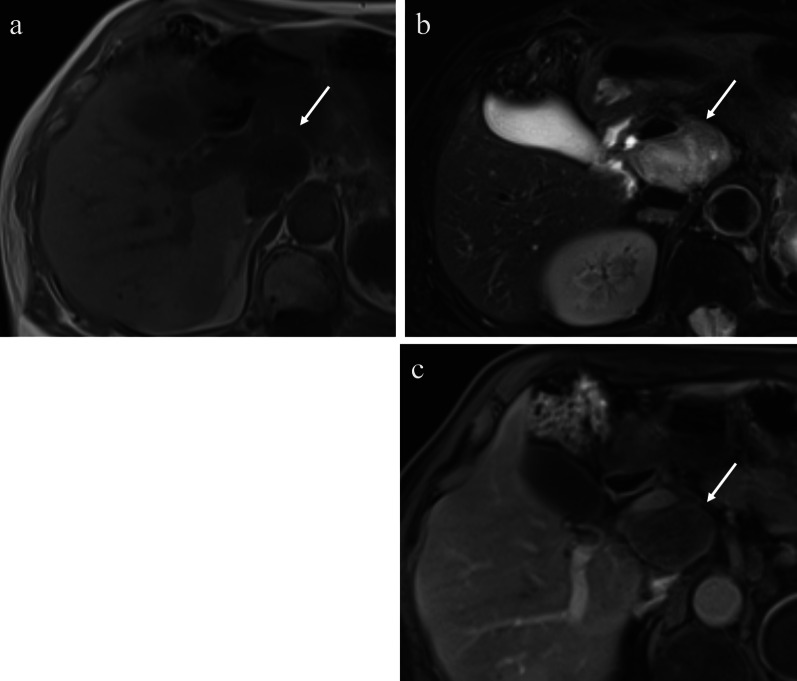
Abdominal magnetic resonance imaging findings. a The tumor (white arrow) showed hypointensity on T1-weighted images. b The tumor (white arrow) showed mixed hypointensity and hyperintensity on T2-weighted fat-suppression images. c Abdominal contrast-enhanced MRI revealed that the peripheral margin of the tumor (white arrow) was enhanced in the arterial phase. *MRI* magnetic resonance imaging
